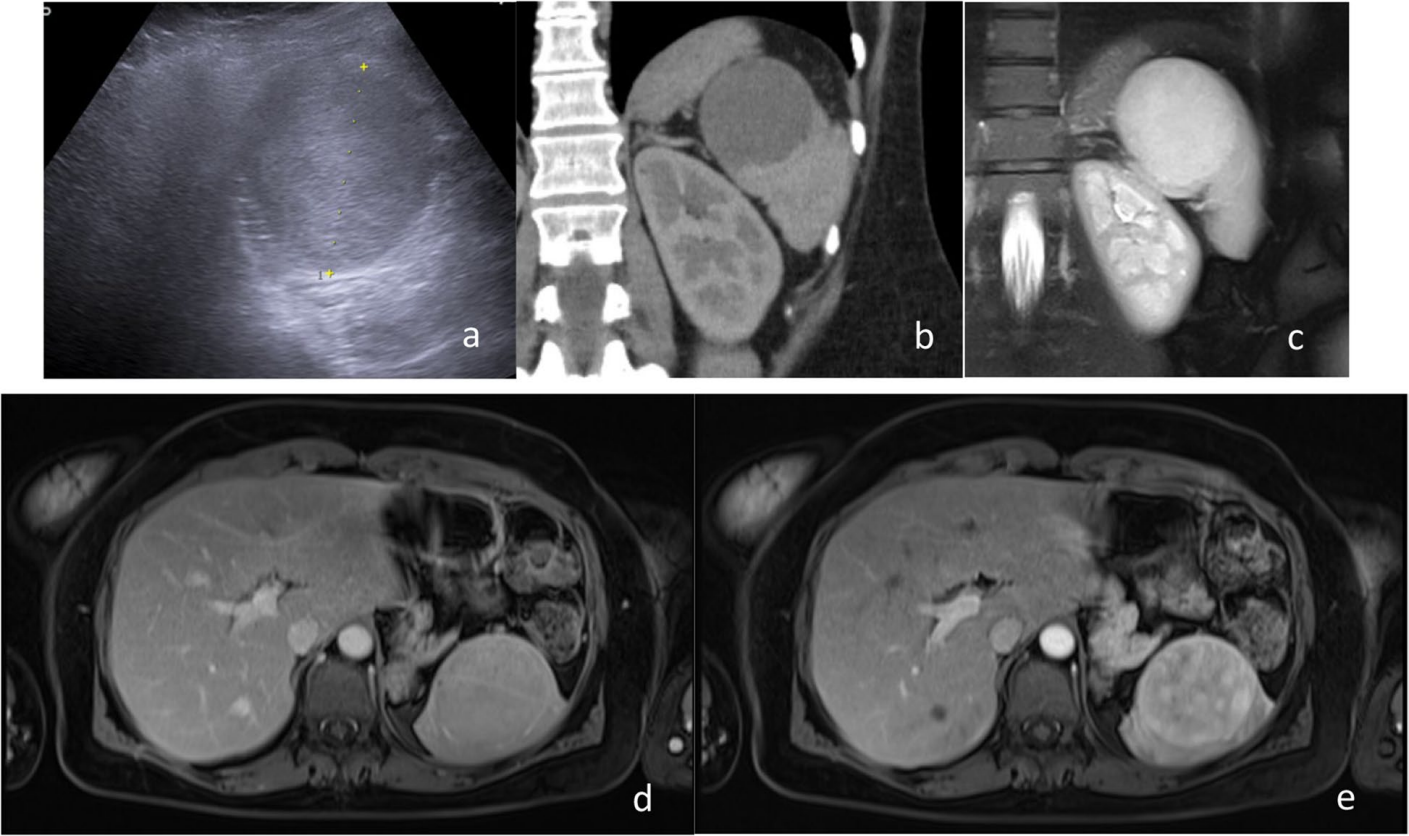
a A well-defined, round, mostly hyperechoic lesion is noted on the upper lobe of the spleen via ultrasound. b The lesion shows early, peripheral, nodular enhancement on CT and is measured (6,6 × 6,3 × 5,6) cm. c the splenic hemangioma shows T2-weighted hyperintensity on MRI. d The mass shows early peripheral enhancement and e late central enhancement on MRI T1-weighted post-gadolinium images
